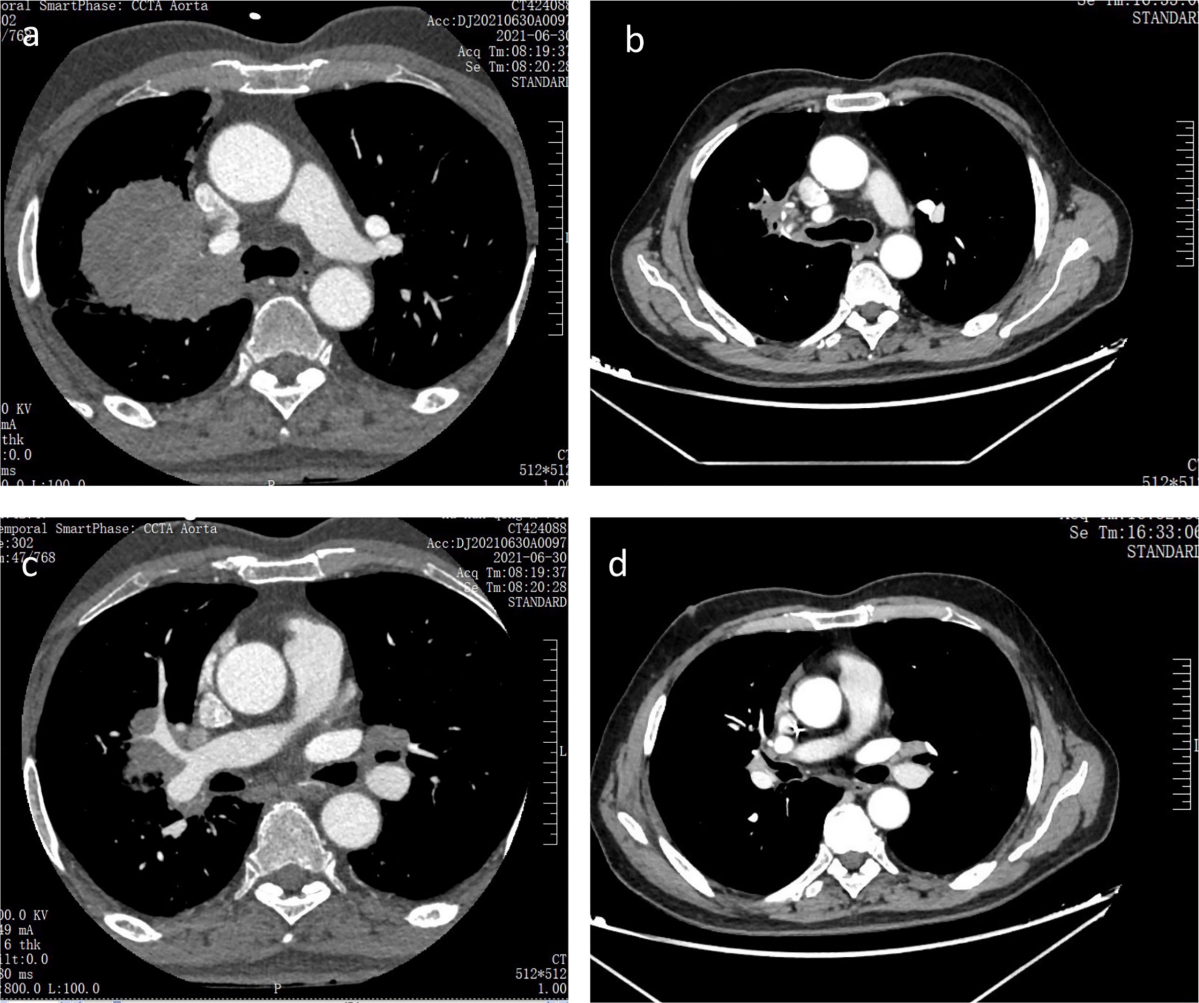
Chest CT scans before and after two cycles of anti-PD-1 immunotherapy combined with anti-angiogenesis therapy and chemotherapy (A, B) Chest CT scans of primary tumor focus in the right lung before and after two cycles of anti-PD-1 immunotherapy combined with anti-angiogenesis therapy and chemotherapy respectively; (C, D) Chest CT scans of the metastatic lymph node in left hilar before and after two cycles of anti-PD-1 immunotherapy combined with anti-angiogenesis therapy and chemotherapy respectively.
